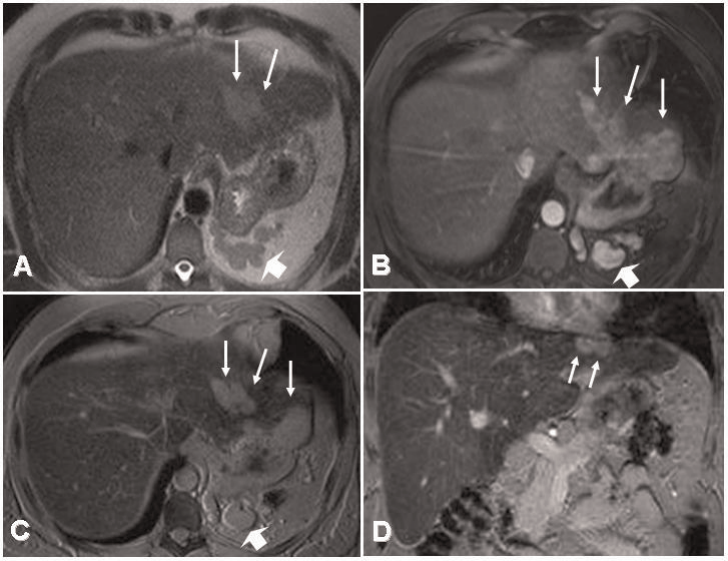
MRI of the liver. T2-weighted single-shot turbo spinecho imaging (HASTE) MRI of the liver shows slightly hyperintense polylobular lesion in the left lobe of the liver, segment II (A, arrows). Multiple nodular structures are identified in the splenic recess indicating recurrent
